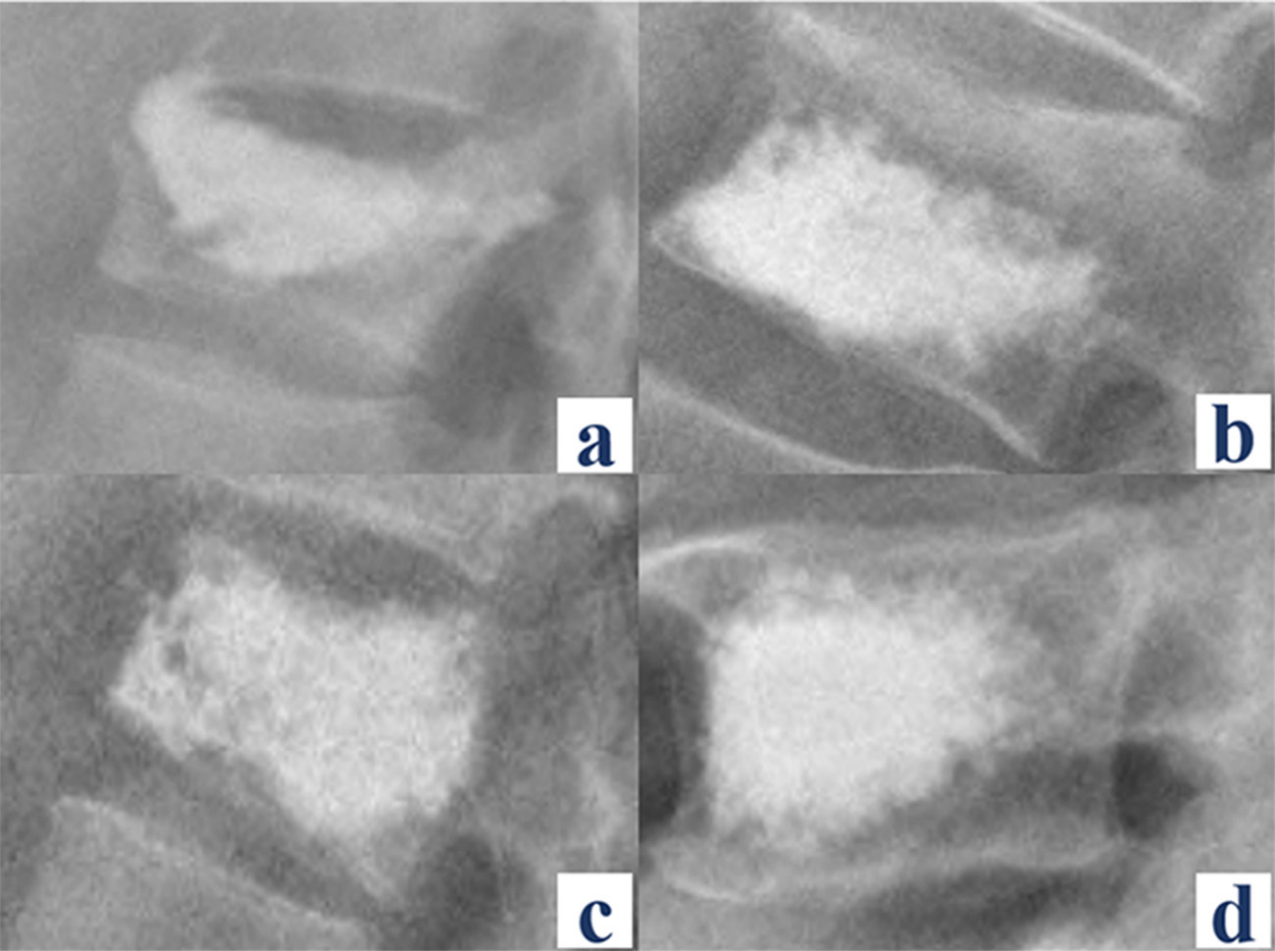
Classification of bone cement form on lateral radiography. (a) Type A – bone cement contacts only the superior endplate of the vertebral body. (b) Type B – bone cement extends only to the inferior endplate of the vertebral body. (c) Type C – bone cement contacts both the superior and inferior endplates of the vertebral body simultaneously. (d) Type D – bone cement does not contact either the superior or the inferior endplate of the vertebral body simultaneously.
